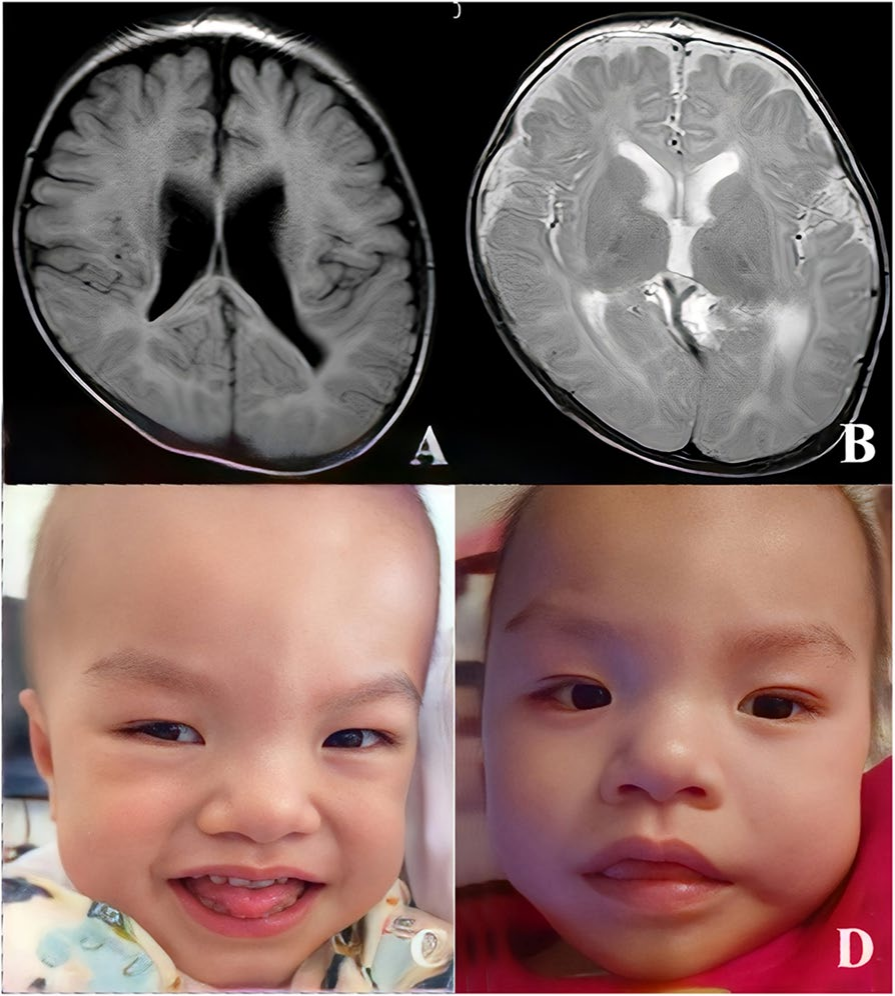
Head MRI Findings and photographs of the dysmorphic features Axial T1-weighted FLAIR (Fluid Attenuated Inversion Recovery) images (A) demonstrated widened anterior cerebral longitudinal fissure and enlarged bilateral ventricles. The subarachnoid space in the anterior part of the cerebral hemisphere was also widened. Axial T2-weighted TSE (Turbo Spin Echo) images(B) demonstrated the same imaging presentations. The patient had special facial feature including tented upper lip, exaggerated Cupid’s bow, arched eyebrows, macroglossia, hypertelorism and open mouth (C & D)
